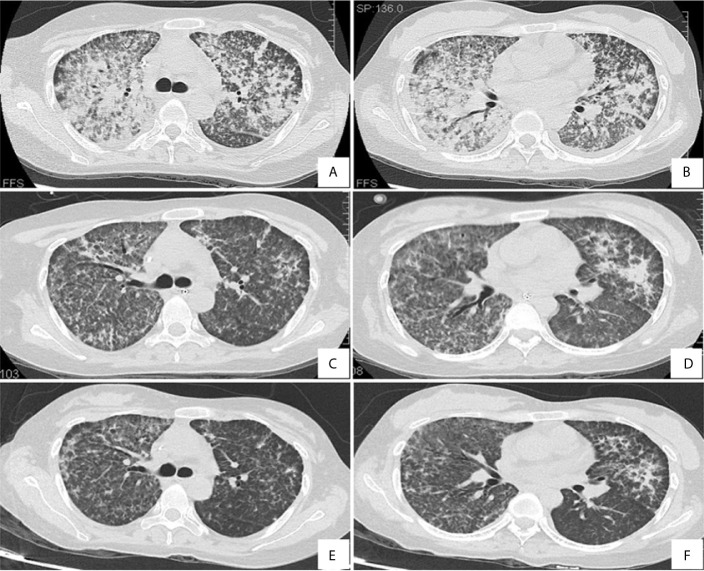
Chest computed tomography scan on day 2 (A, B), day 8 (C, D) and day 17 (E, F).
